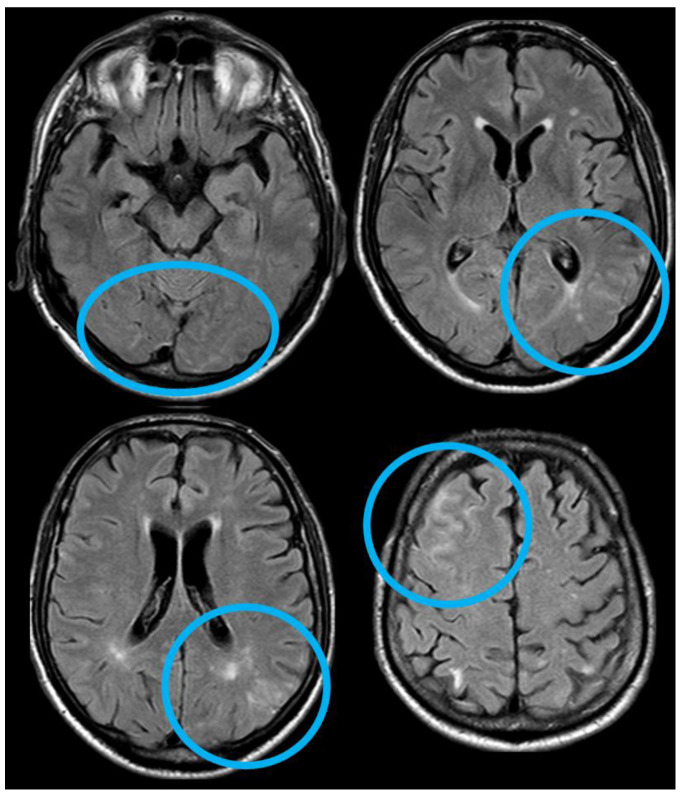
Brain MRI (axial FLAIR) showing spontaneous sulcal hyperintensity involving several sites, in particular, the occipital pole on both sides (left > right), the left temporal-occipital transition, the left parietal lobe, and the right anterior frontal lobe (blue circles).
